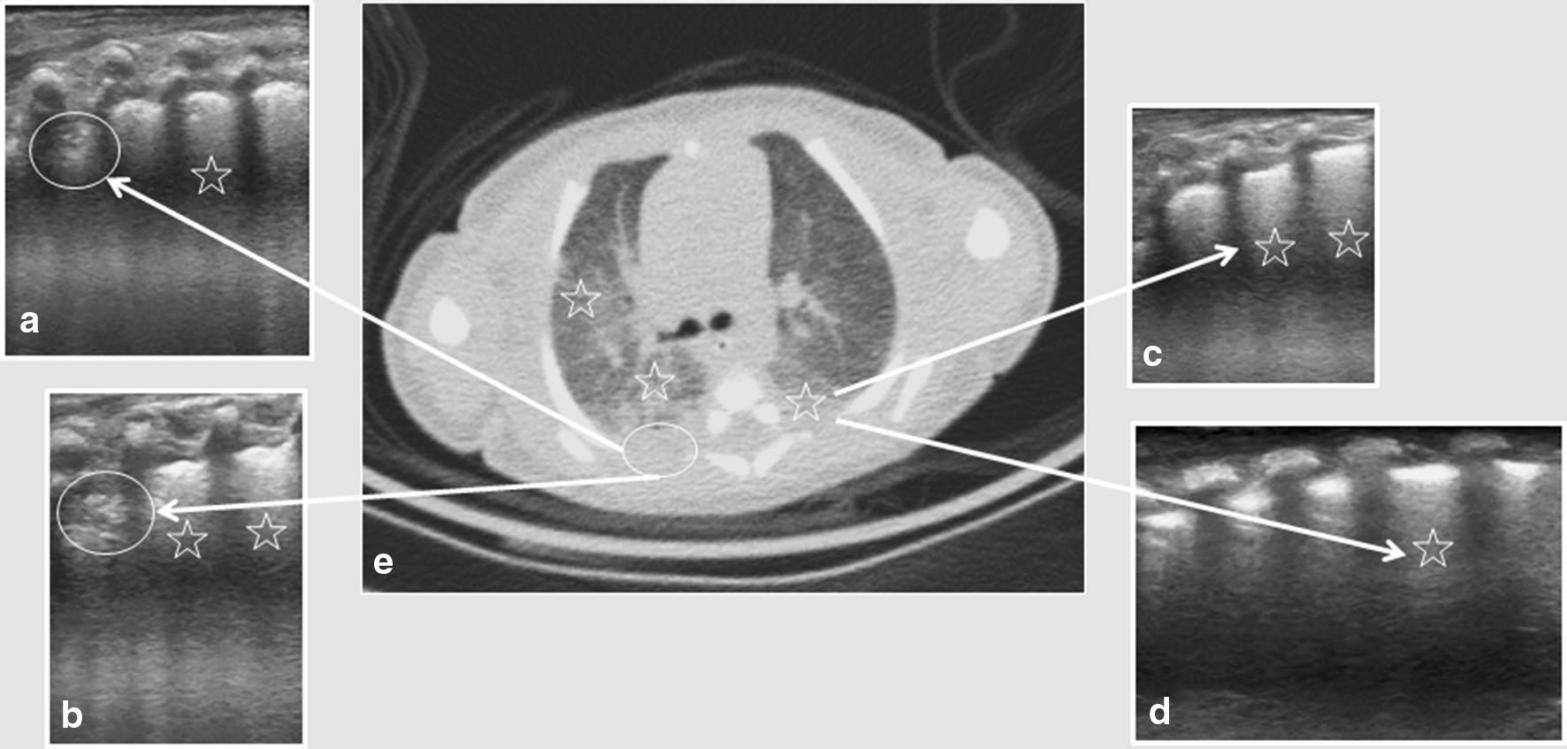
Thirteen-day-old male preterm newborn, presented with hypoxemia, tachypnea and high D-dimer level. Lung US (a–d) showed irregularities of the pleural line and small subpleural consolidations in the posterior right lung field (circles in a, b). Confluent B-lines (stars in a–d) were also present in the bilateral posterior and inferior lung fields corresponding to the chest CT (e) ground-glass pattern (stars in e). Chest CT showed good correlation with lung ultrasound findings (arrows in a–e)
